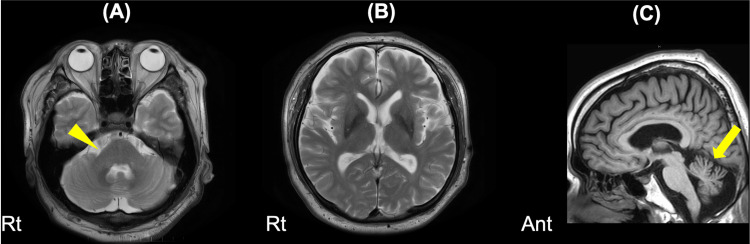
MRI with contrast showing cross sign and cerebellar atrophy. (A) T2-weighted images on axial views are shown. The yellow arrowhead indicates a possible finding of a cross sign in the pons. (B) Atrophy of the putamen and linear T2 hyperintensity in the lateral portion of the putamen were not observed. (C) T1-weighted image on sagittal view is shown. Cerebellar atrophy may be present (yellow arrow).
